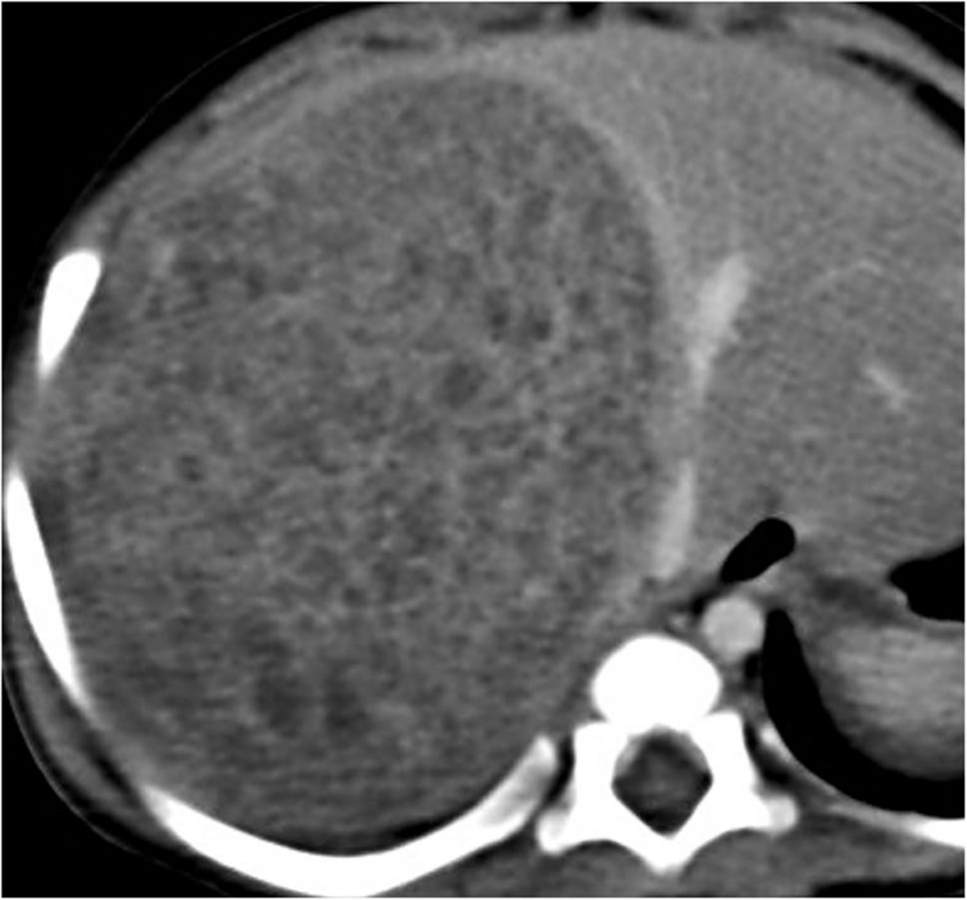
Axial portal venous phase CT abdomen of a 15-month-old boy with mesenchymal hamartoma shows a well-defined lesion with multiple smaller cysts, giving a “sieve-like” appearance
